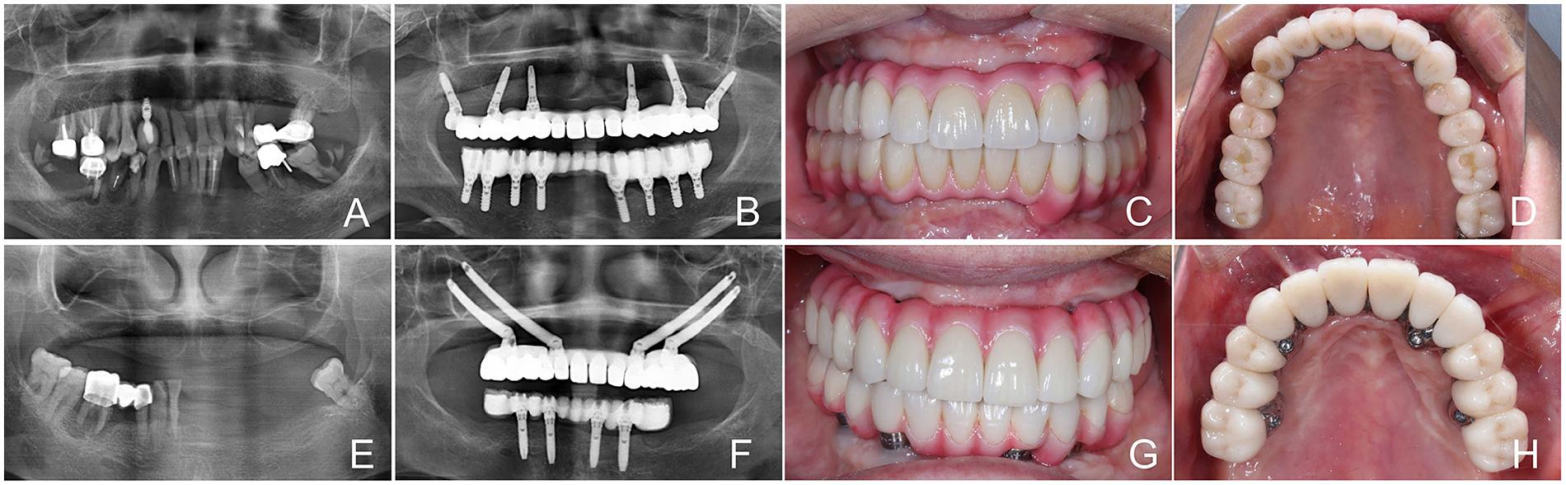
Representative cases of CIFR (A–D) and ZIFR (E–H). (A) Preoperative panoramic radiograph of a CIFR case; (B) panoramic radiograph after prosthesis delivery; (C) intraoral photograph; (D) intraoral photographs of the occlusal surface; (E) preoperative panoramic radiograph of a ZIFR case; (F) panoramic radiograph after prosthesis delivery; (G) intraoral photograph; and (H) intraoral photographs of the occlusal surface.
